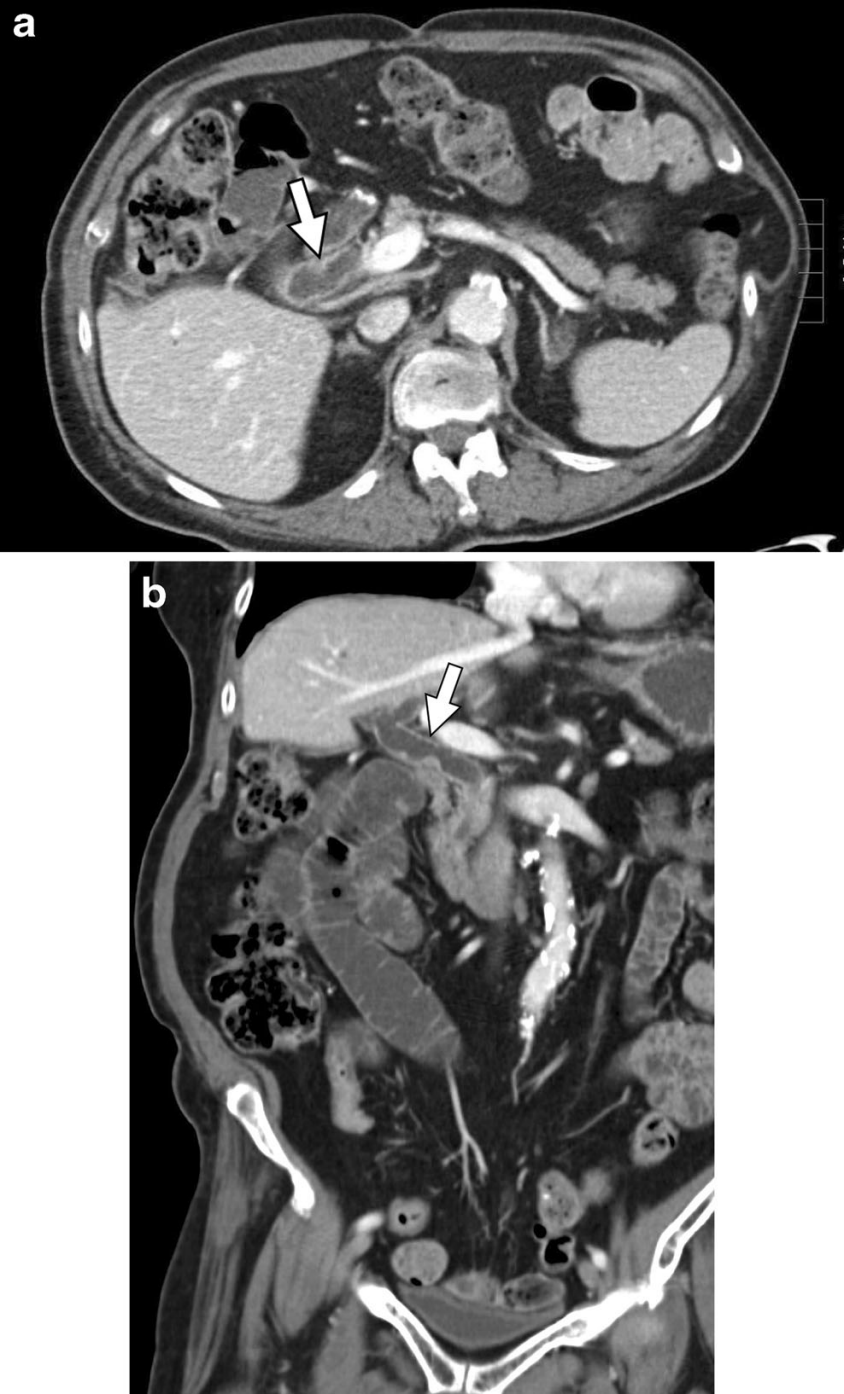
An 81-year-old female with stage IV recurrent squamous NSCLC treated with carboplatin, gemcitabine tarceva, and abraxane. a, b Axial and coronal contrast-enhanced CT images, respectively, demonstrate a mildly dilated common bile duct (*arrows*) with thickened enhancing walls consistent with biliary epithelial irritation
